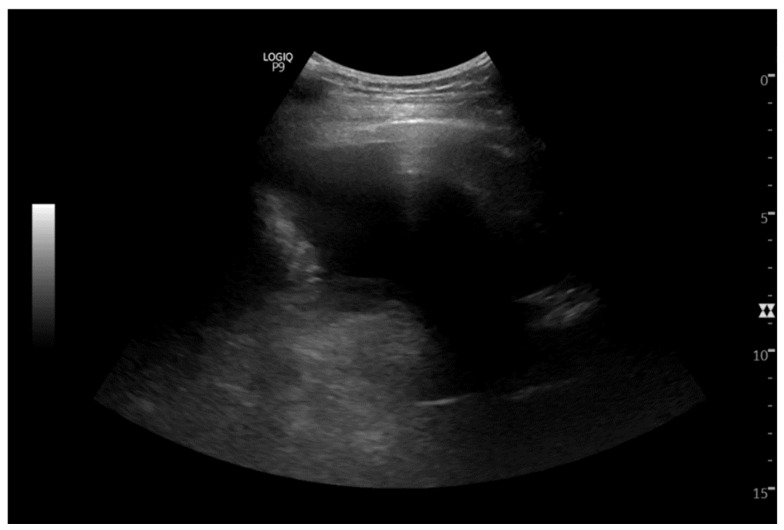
Ultrasound demonstrating a moderate left pleural effusion. Ultrasound-guided thoracocentesis was performed.
